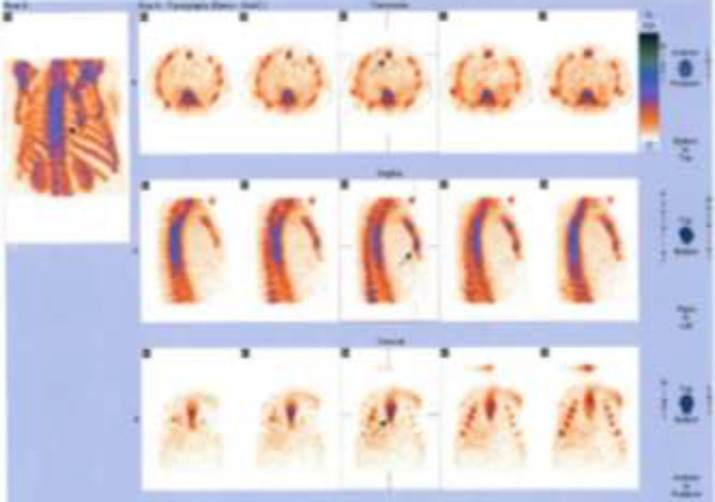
SPECT imaging of the patient displayed in figure 1. The arrow in the picture shows the metastasis site in the sternum
